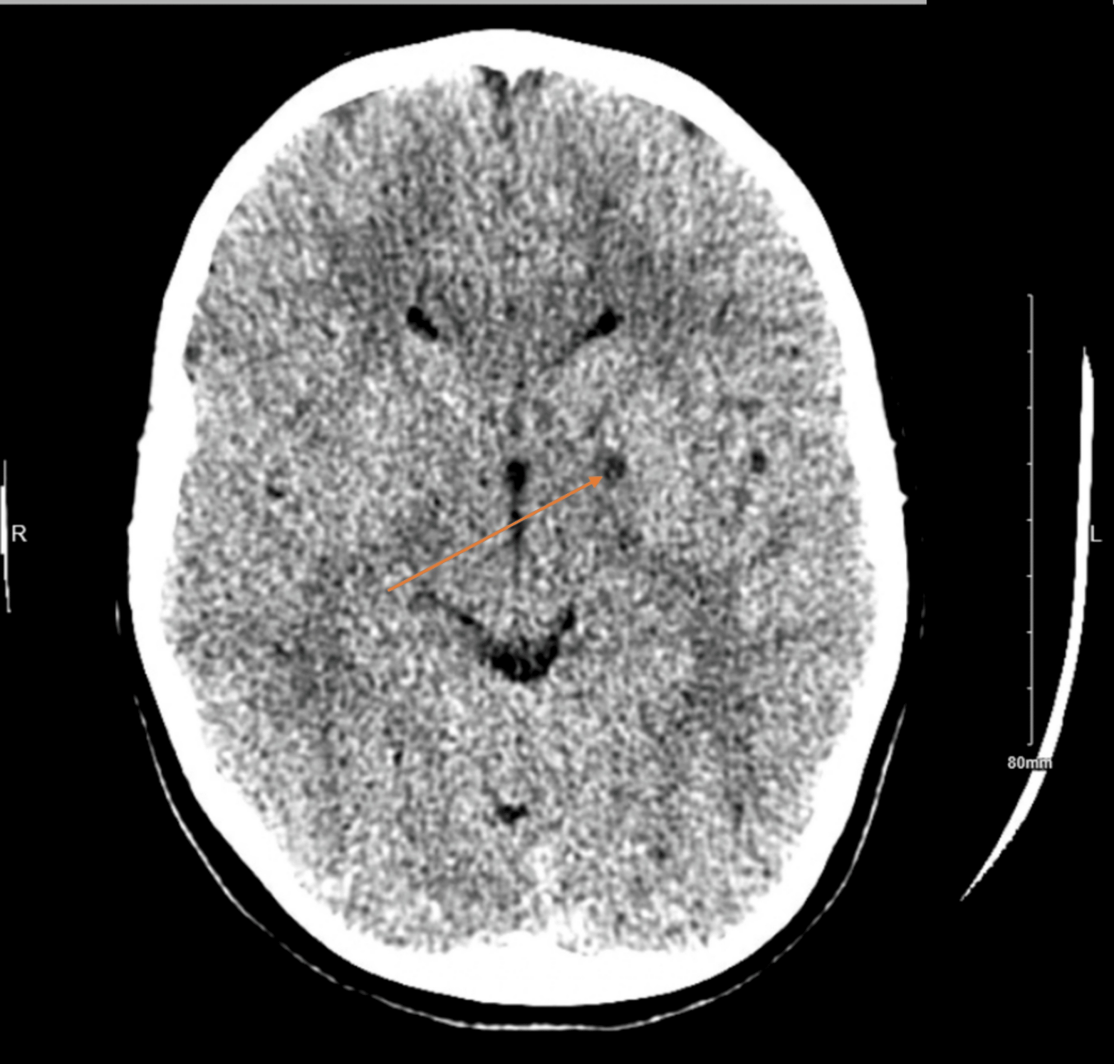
Non-contrast axial brain CT demonstrating a small, focal hypodensity within the left basal ganglia (arrow), measuring approximately 4 mm and reported as likely representing a prominent perivascular space, with no associated mass effect or hemorrhage.
